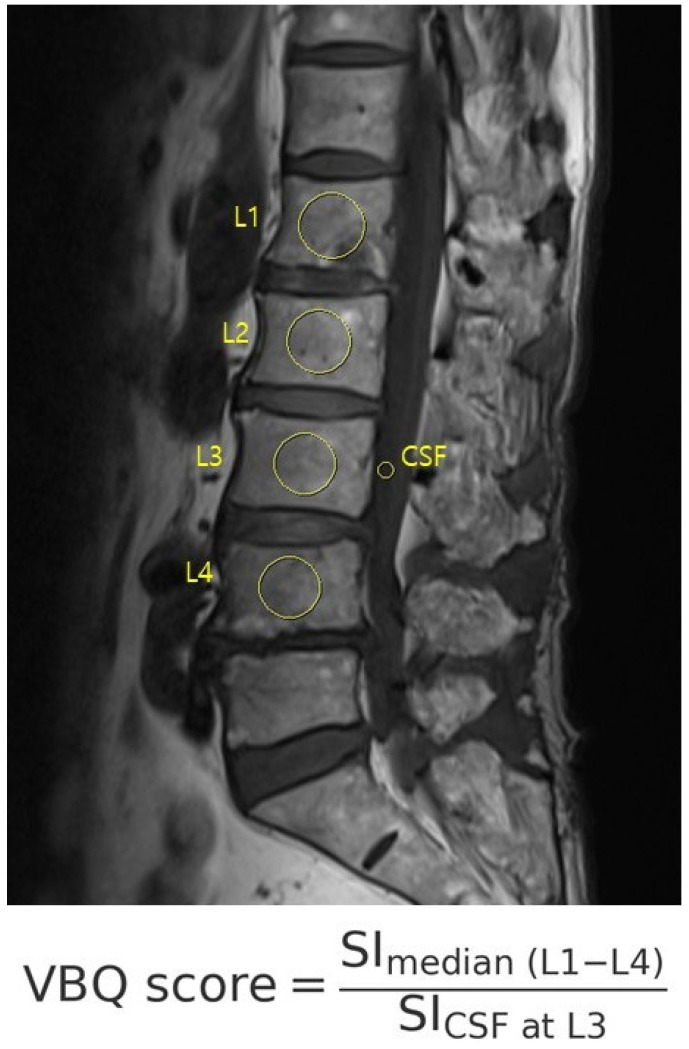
The VBQ score was calculated on T1-weighted MRI as the ratio of the vertebral body to CSF signal intensity, using manually placed ROIs from L1 to L4 and CSF at L3.
